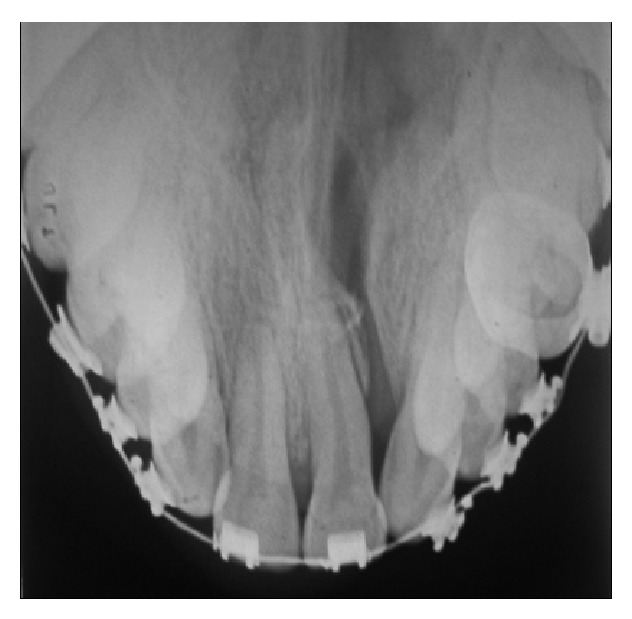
Occlusal radiograph. You can see the cleft through the palate compromising alveolar ridge and hard palate.
